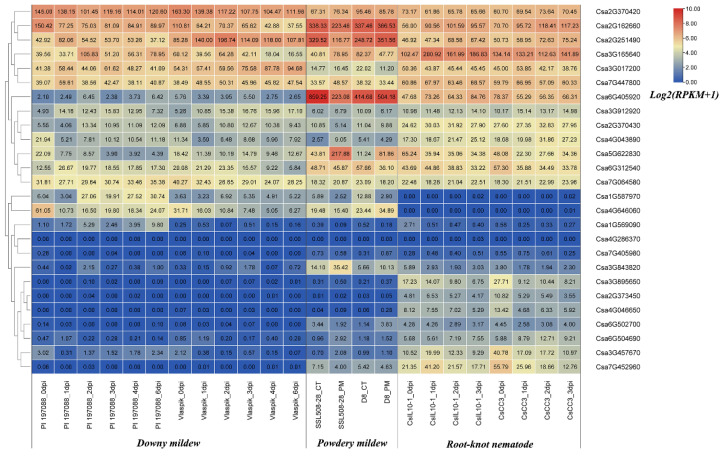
Expression profiles of cucumber GATA genes in response to various biotic stress treatments including downy mildew, powdery mildew and root-knot nematode. PI 197088 is the downy mildew-resistant cucumber plant; Vlaspik is the downy mildew-susceptible cucumber plant; dpi means the days post inoculation. SSL508-28 is the powdery mildew-resistant cucumber plant; D8 is the powdery mildew-susceptible cucumber plant; CT is the control plant; PM is the abbreviation of powdery mildew. CsIL10_1 is the root-knot nematode resistant cucumber plant, CsCC3 is the root-knot nematode susceptible cucumber plant. The RPKM values of GATA genes were transformed by *log2(RPKM+1)*. The data in the boxes indicated original RPKM values. The red and blue colors represented the higher and lower relative expression levels, respectively.
